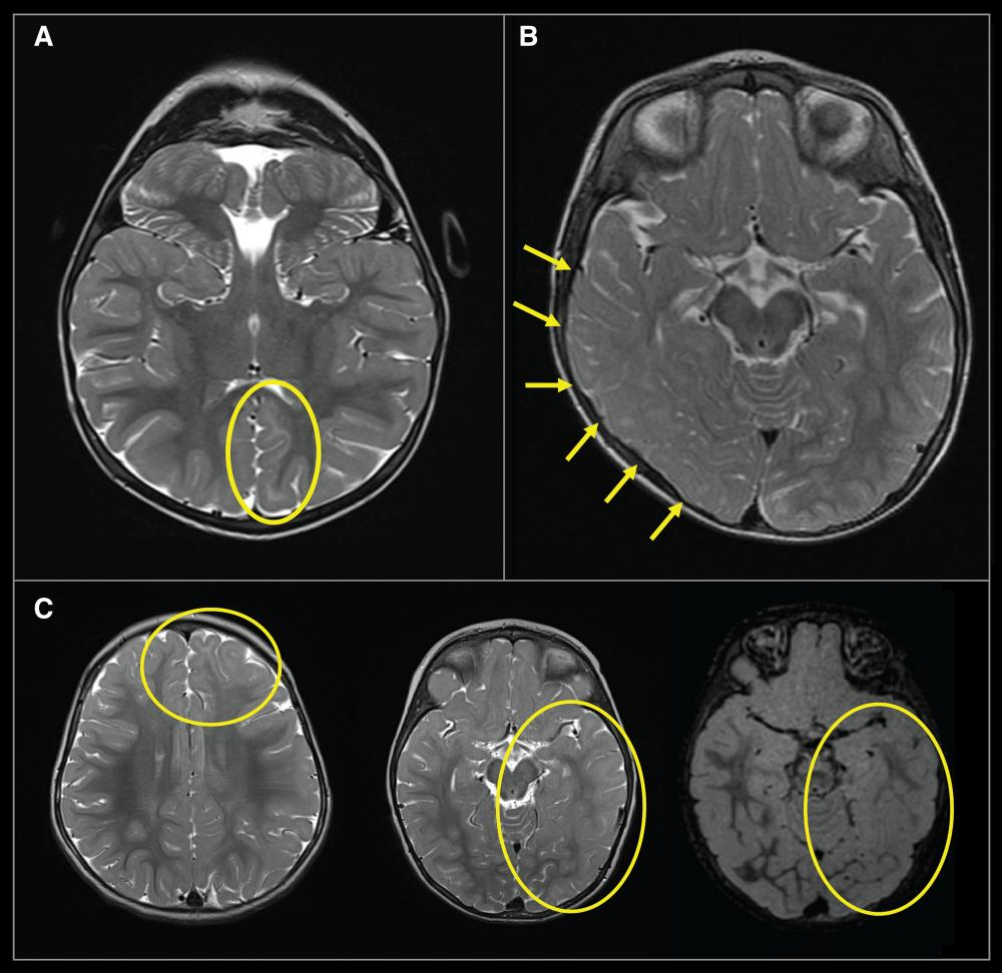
Representative MRI scans from this cohort demonstrating features of MOGHE subtypes 1 and 2. (A) Shows axial view MRI of individual AA0190-01. Evidence of increased fluid attenuated inversion recovery (FLAIR) signal at the corticomedullary junction (consistent with MOGHE subtype 1) is indicated in the yellow circled area. (B) Shows axial view MRI of individual AA01391-01. Arrows indicate reduced corticomedullary differentiation because of increased signal of the adjacent white matter in the right temporal lobe (MOGHE Subtype 2). (C) Shows three axial slices from individual AA0228-01 with features of MOGHE subtype 2 in the posterior region, and subtype 1 in anterior regions, indicated by yellow circles.
